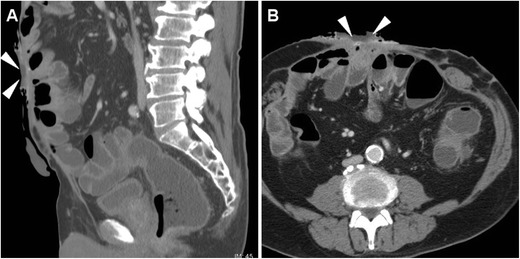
High-output ECF in a 69-year-old man with history of repeated surgeries to manage jejunal and duodenal perforations from Zollinger-Ellison syndrome, followed by hemicolectomy, ileostomy, and colostomy because of ischemic bowel necrosis. At CT, the wide draining EFO corresponded to a 2-cm hypoattenuating discontinuity (arrowheads) in the thickened skin, closely adherent to distended bowel loops with air-fluid levels consistent with postoperative ileus
